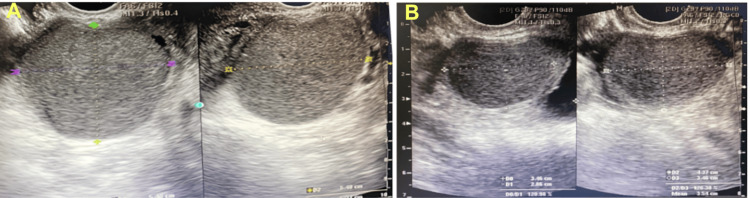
Transvaginal ultrasound findings: (A) A hypoechoic mass (54 × 53 × 54 mm) in the left ovary prior to medical therapy; (B) A hypoechoic mass (45 × 35 × 29 mm) in the right ovary prior to medical therapy
